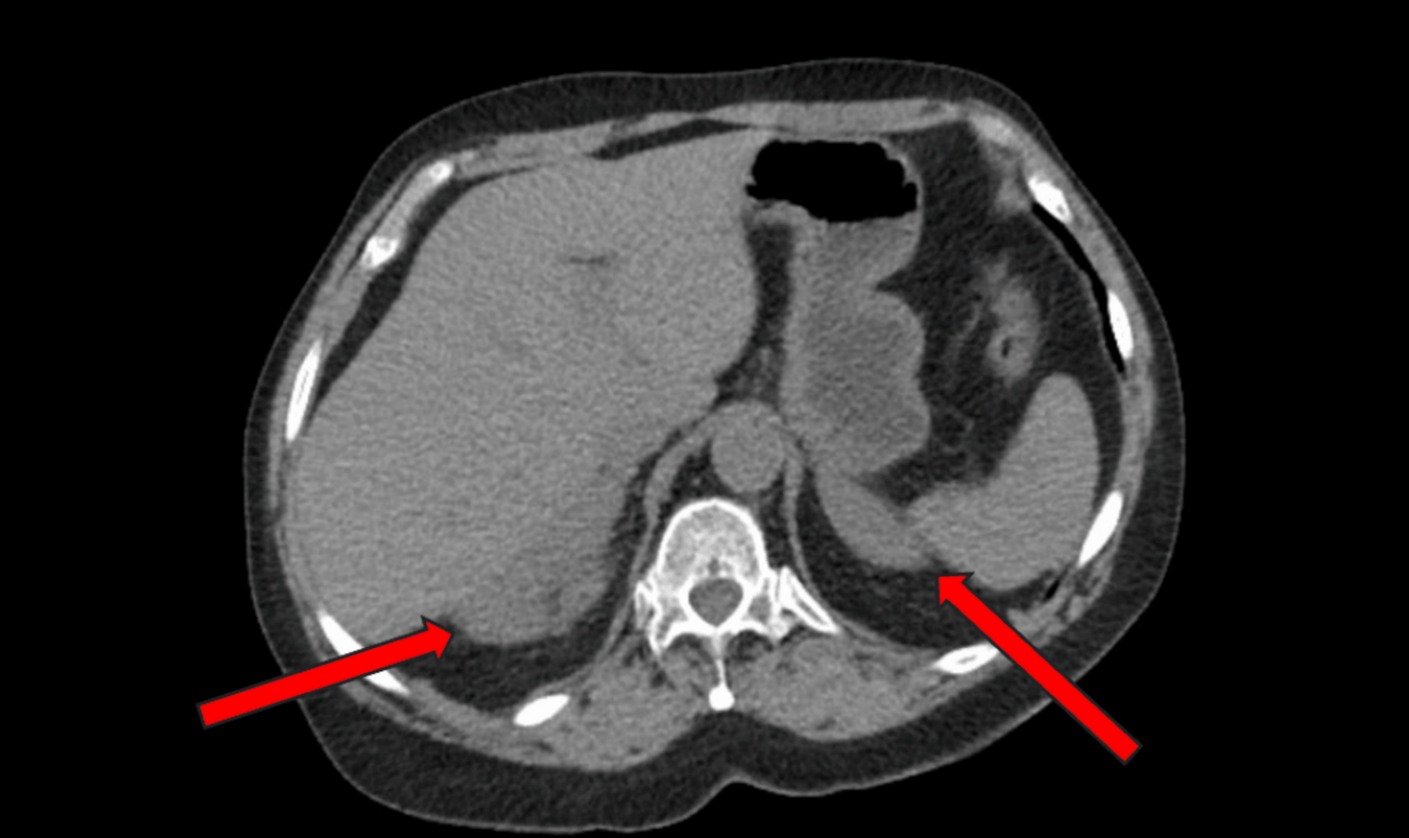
CT abdomen and pelvis demonstrating bilateral adrenal gland enlargement (indicated by red arrows). The adrenal glands appear diffusely enlarged without discrete masses, consistent with adrenal hyperplasia.
